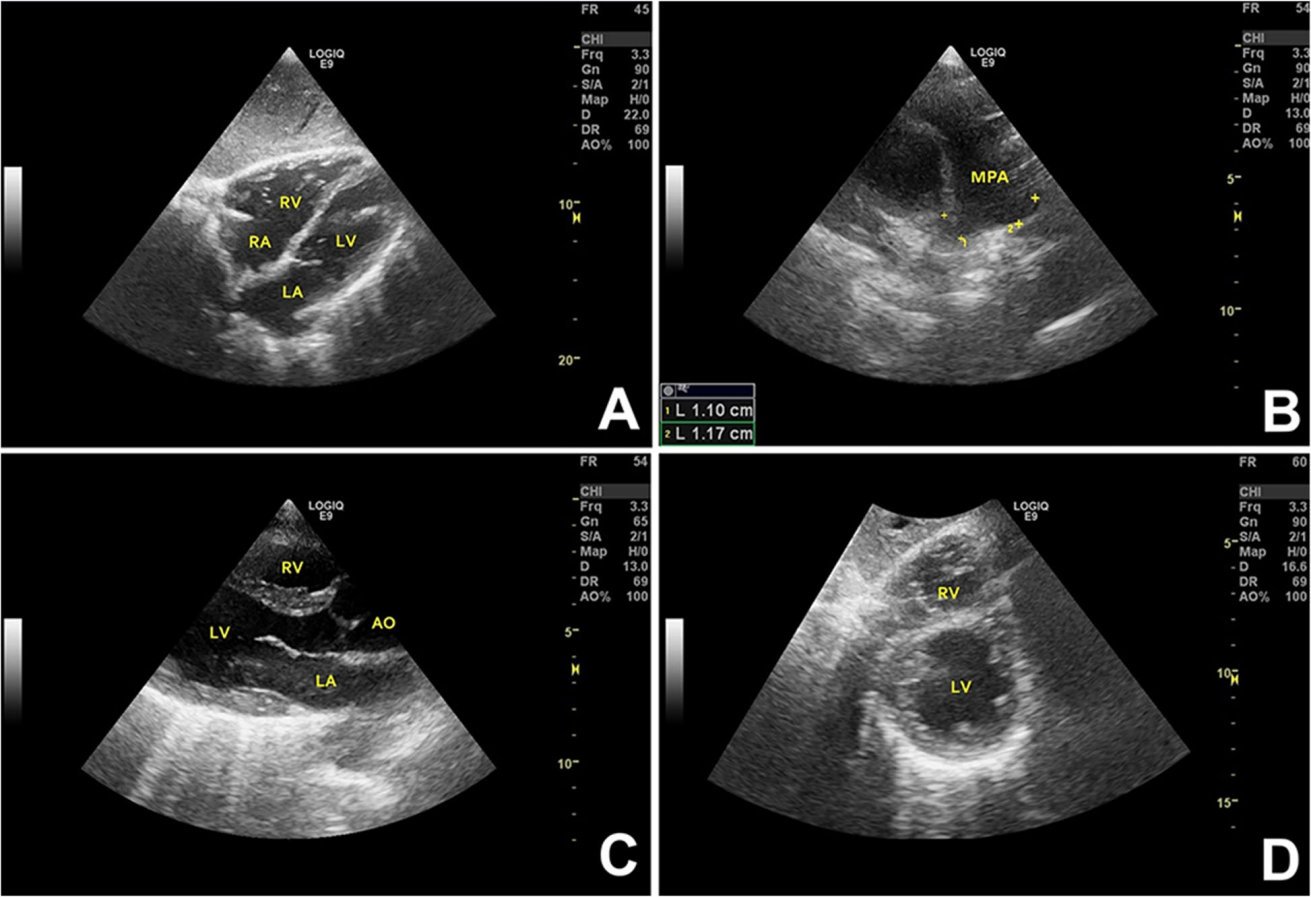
Echocardiography of the patient after one month of treatment (A-D). Apical four-chamber view showing normal RA and RV (A); Short-axis view of the heart showing the normal main pulmonary artery (MPA) and its branches (B); Long axial section of LV showing the normal range of left atrial (LA) and LV size (C); Left ventricle short-axial view showing normal chamber wall thickness; LV end-diastolic wall thickness is 9 mm (D)
